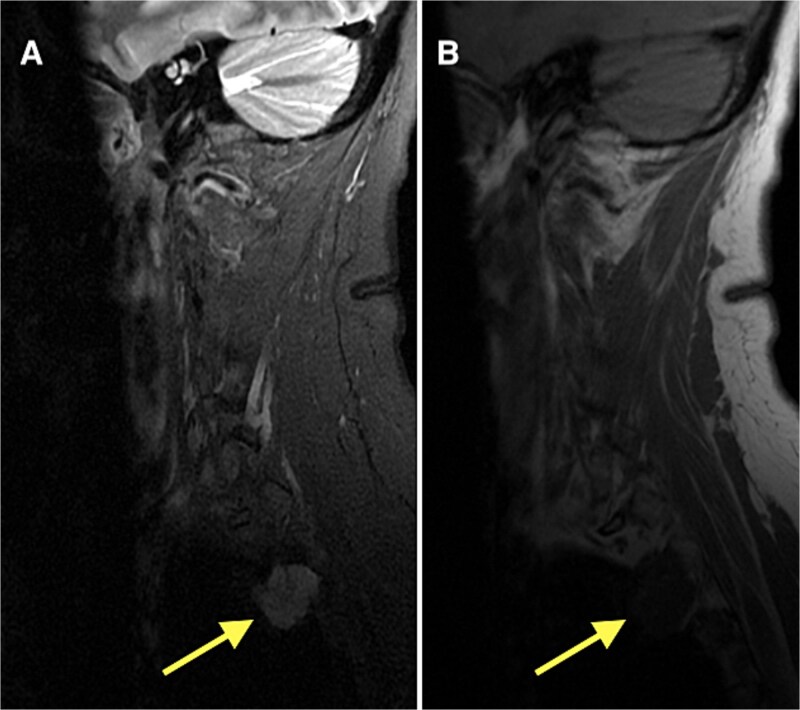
MRI of the thoracic spine demonstrating a lobulated T1-hypointense (B), T2-hyperintense lesion (A) at the lateral margin of the right T2–T3 neural foramen, measuring 2.3 × 2.2 cm (anteroposterior × transverse).
